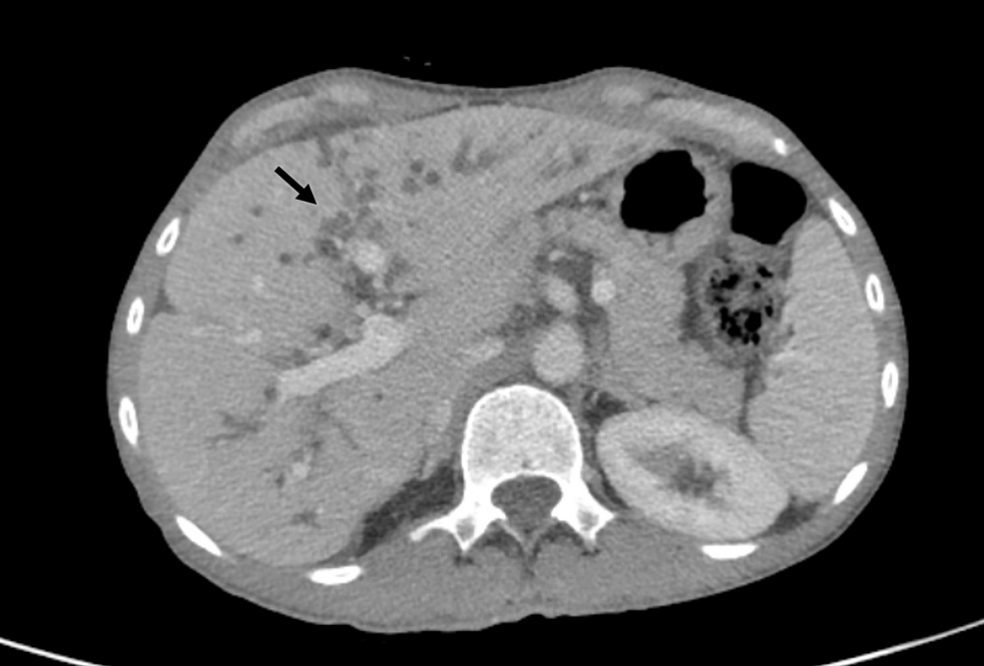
Three-phasic CT scan in the portovenous phase of a 34-year-old female, which is a known case of PSC, referred for liver transplantation. The beaded appearance of biliary tree is noted. PSC: primary sclerosing cholangitis
